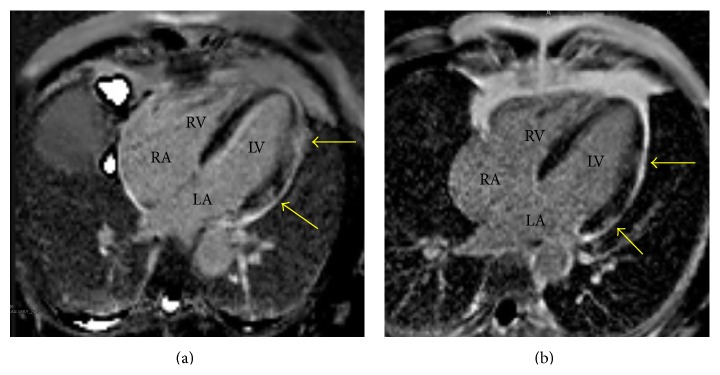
Cardiac magnetic resonance image (four-chamber view). (a) Presentation: patchy areas of late gadolinium enhancement (LGE) were detected transmurally in the mid lateral left ventricular (LV) wall and in the midwall with extension to the subepicardial in lateral and anterior LV walls near the base consistent with diffuse inflammation in a noncoronary distribution (arrows). (b) 6 weeks after presentation: markedly reduced LGE (arrows) consistent with improvement in inflammation without significant residual fibrosis. LV: left ventricle, RV: right ventricle, LA: left atrium, and RA: right atrium.
